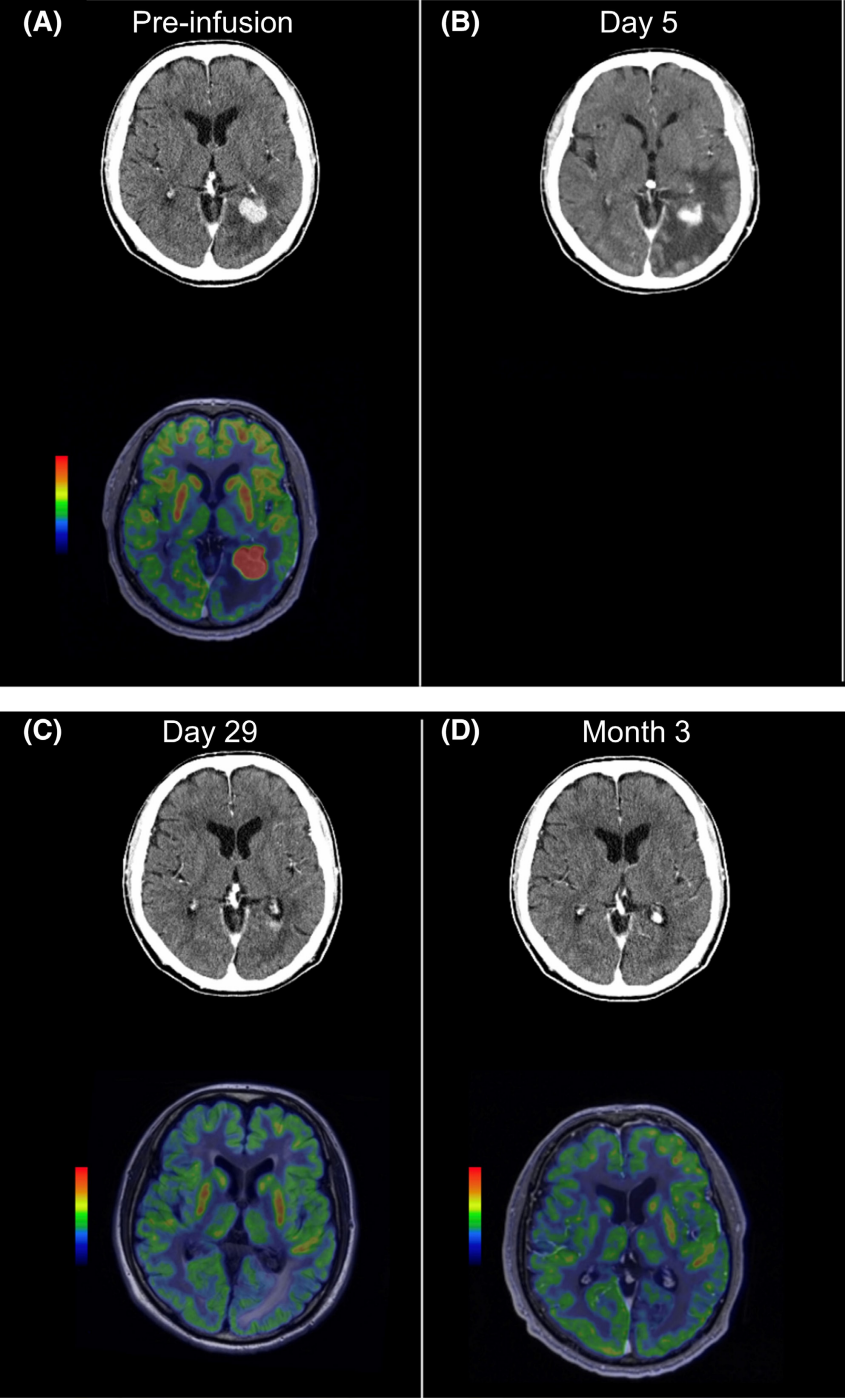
Patient with secondary CNS lymphoma. (A) The patient received two cycles of high‐dose methotrexate to prevent rapid progression of the brain mass lesion, with a response of stable disease (pre‐infusion). (B) A computed tomography scan on day 5 showed decrease of the CNS lesion while peritumoral cerebral edema was observed. (C) To improve the cerebral edema, high‐dose steroids (dexamethasone 20 mg/day every 6 h for 2 days and tapered) were started. After the initiation of steroid, the patient's symptoms rapidly improved, and he achieved a complete metabolic response per investigator assessment on day 29. (D) The complete metabolic response persisted for 3 months after infusion (day 102). CNS, central nervous system.
